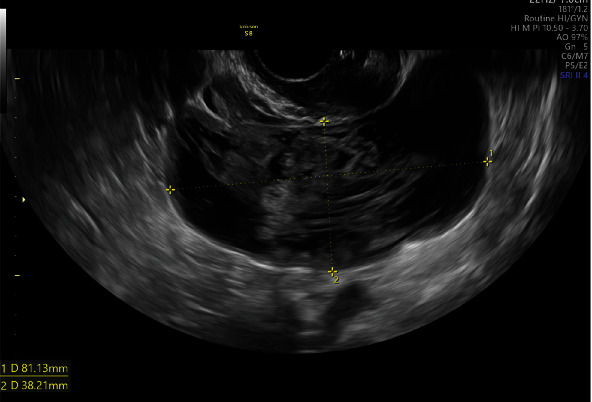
The transvaginal sonography revealed a right adnexal complex mass measuring 8.1 × 3.8 cm that did not resolve after three months of expectant management.
